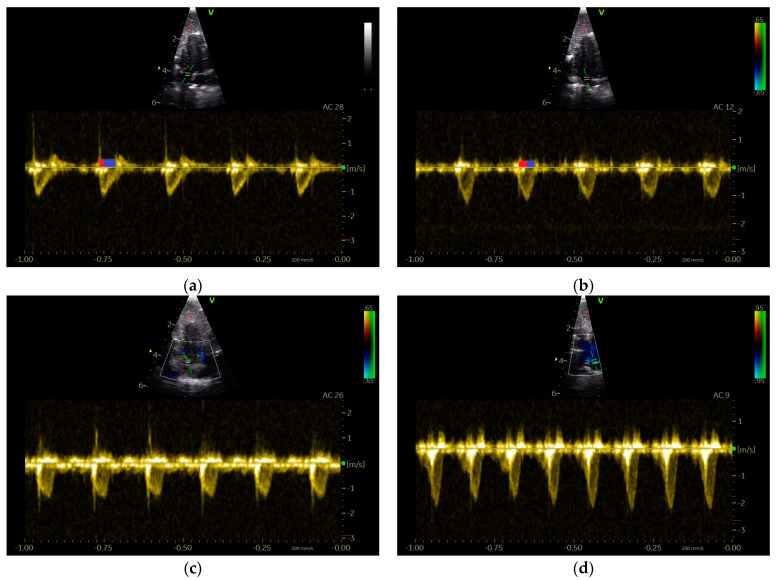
PW Doppler sonographic blood flow profiles of the aorta (a) and pulmonary arteries (b–d) of Congo grey parrots without sonographic evidence of heart disease (GPs from group 1). The acceleration phase of the blood flow is visible in red and the deceleration phase in blue in (a,b). A sample gate is placed in the area of the aortic (a) or pulmonary valve (b–d); AC: angle correction. The colour scale on the right side of the images is calibrated in m s^−1^.
